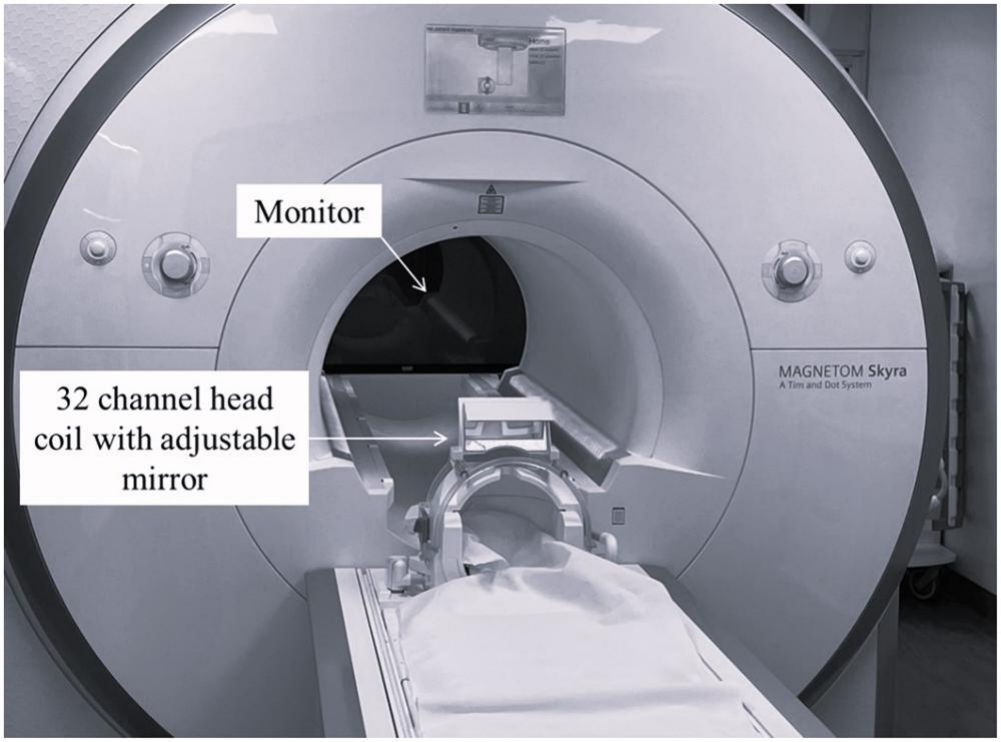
3 T Siemens Magnetom Skyra MRI scanner with a 32-channel head coil.
